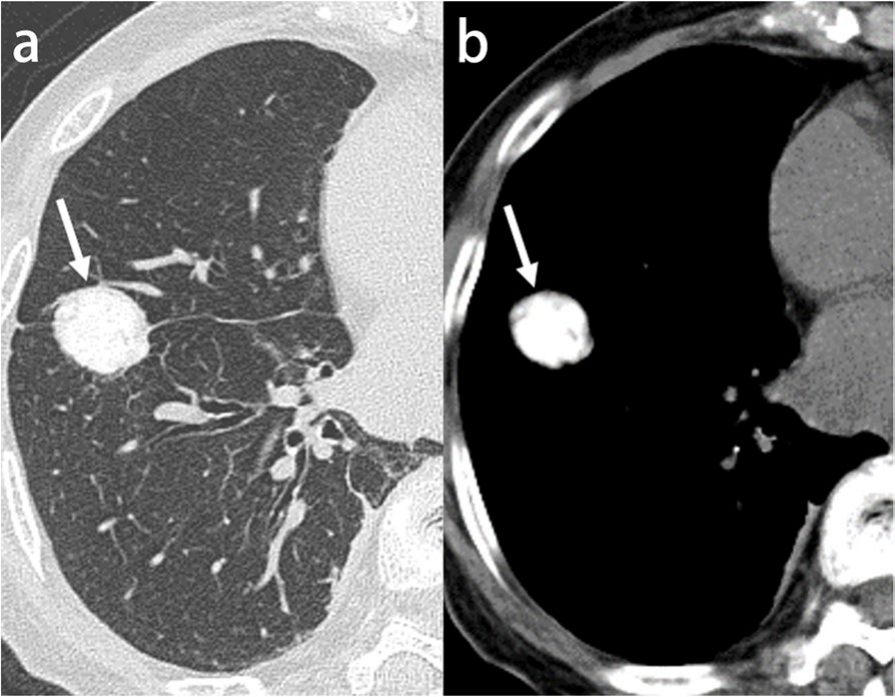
Talc pleurodesis in an 81-year-old woman. a, b CT images show a nodular high-attenuation talc deposit in the interlobar fissure (arrow)
